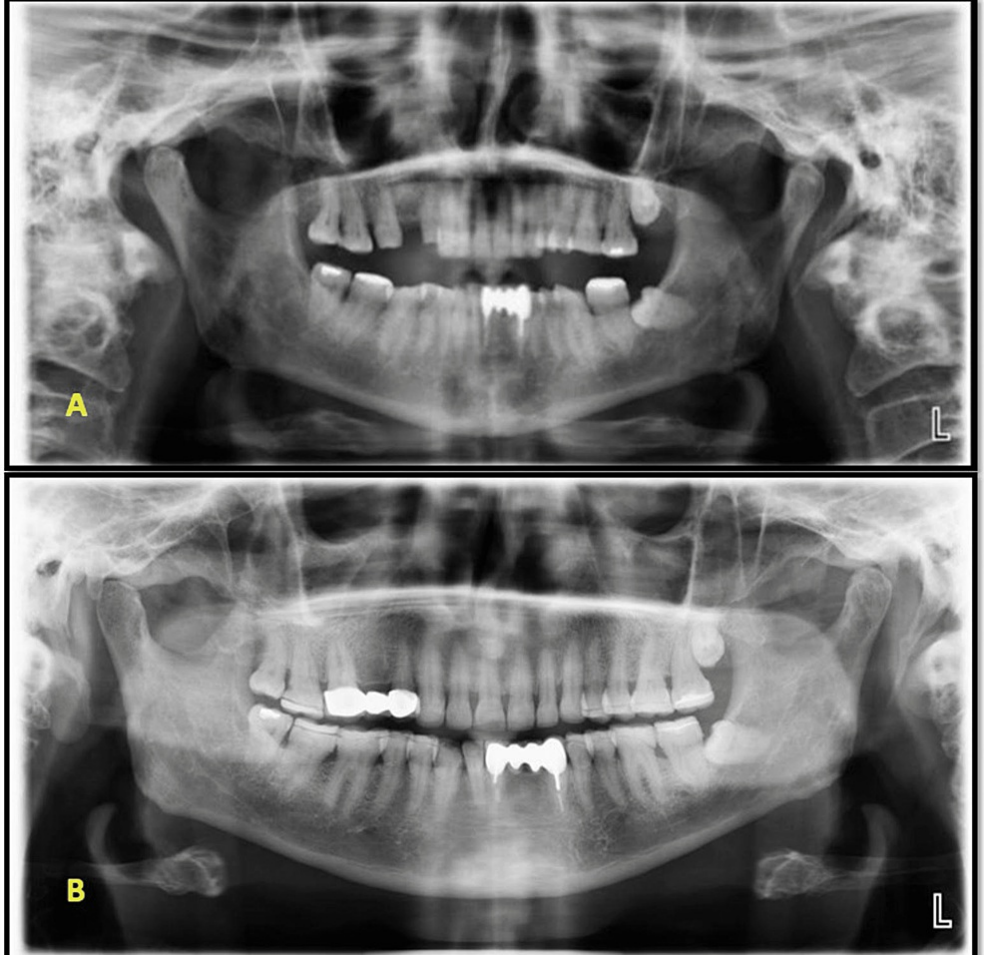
Preoperative and postoperative radiographs A: preoperative radiograph, B: one-year postoperative radiograph
